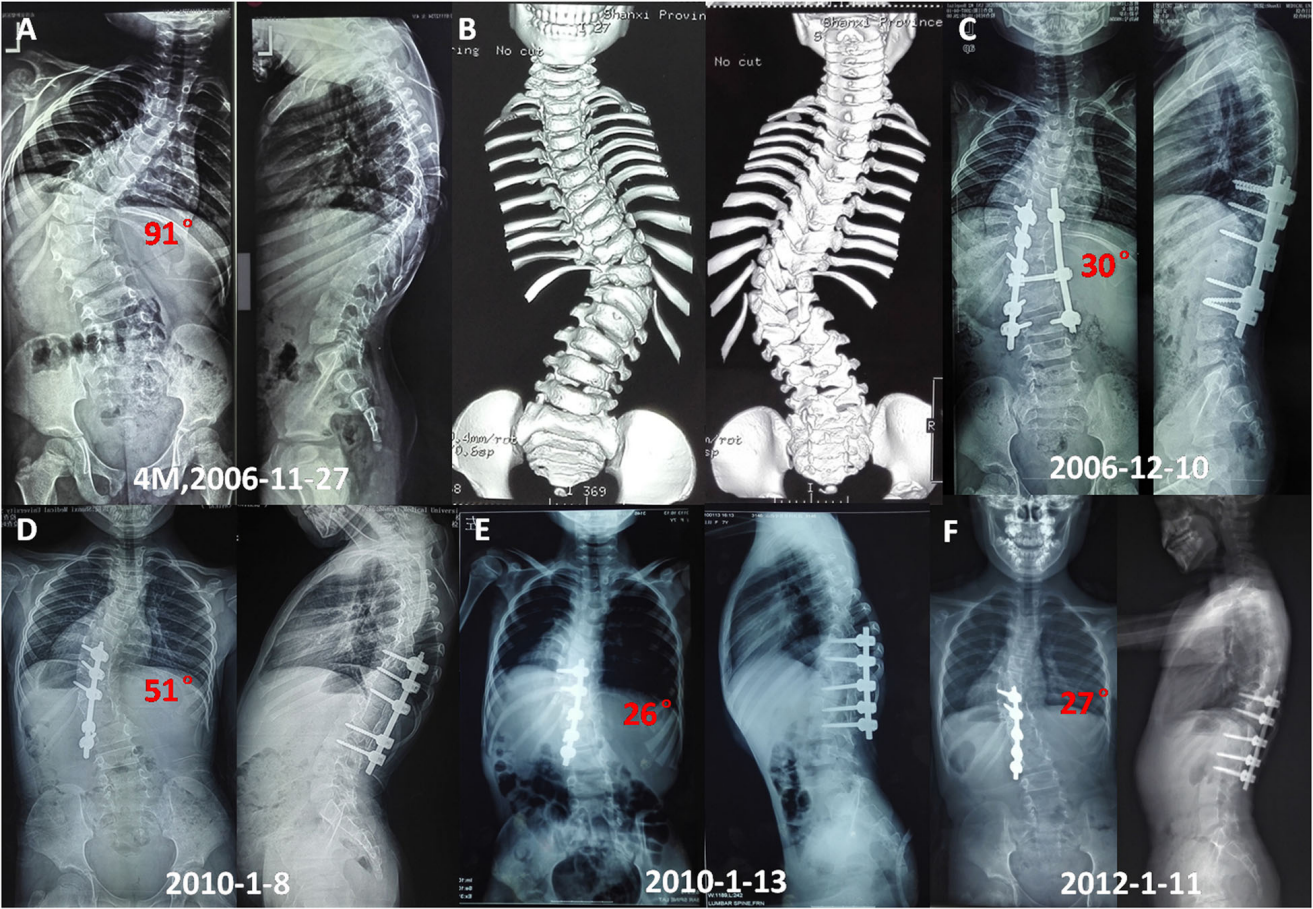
A 4 years old boy with complex CS. Radiographs and 3D CT showed T10, 12 full-segmented hemivertebra with concave contralateral bar, and 9th bifid rib (A,B). He underwent T10 and T12 hemivertebrectomy at initial surgery with short fusion(C). Second T11 Y-shaped osteotomy surgery was performed after 36 months because curve progression. Radiographs images obtained preoperatively, postoperatively and at the latest follow-up 24 months later (D,E,F)
